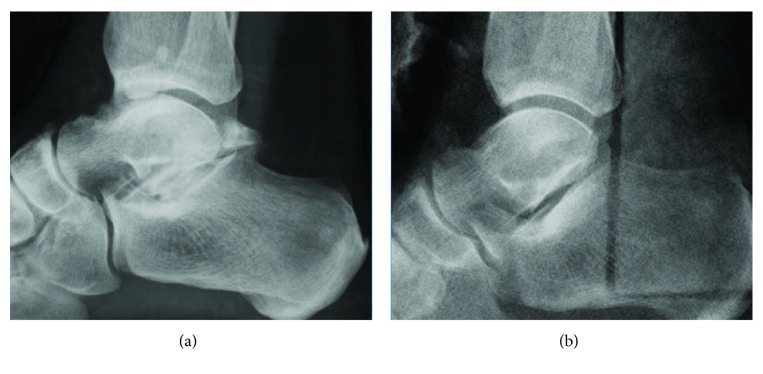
Comparative preoperative (a) and postoperative X-rays with no residual bone (b).
